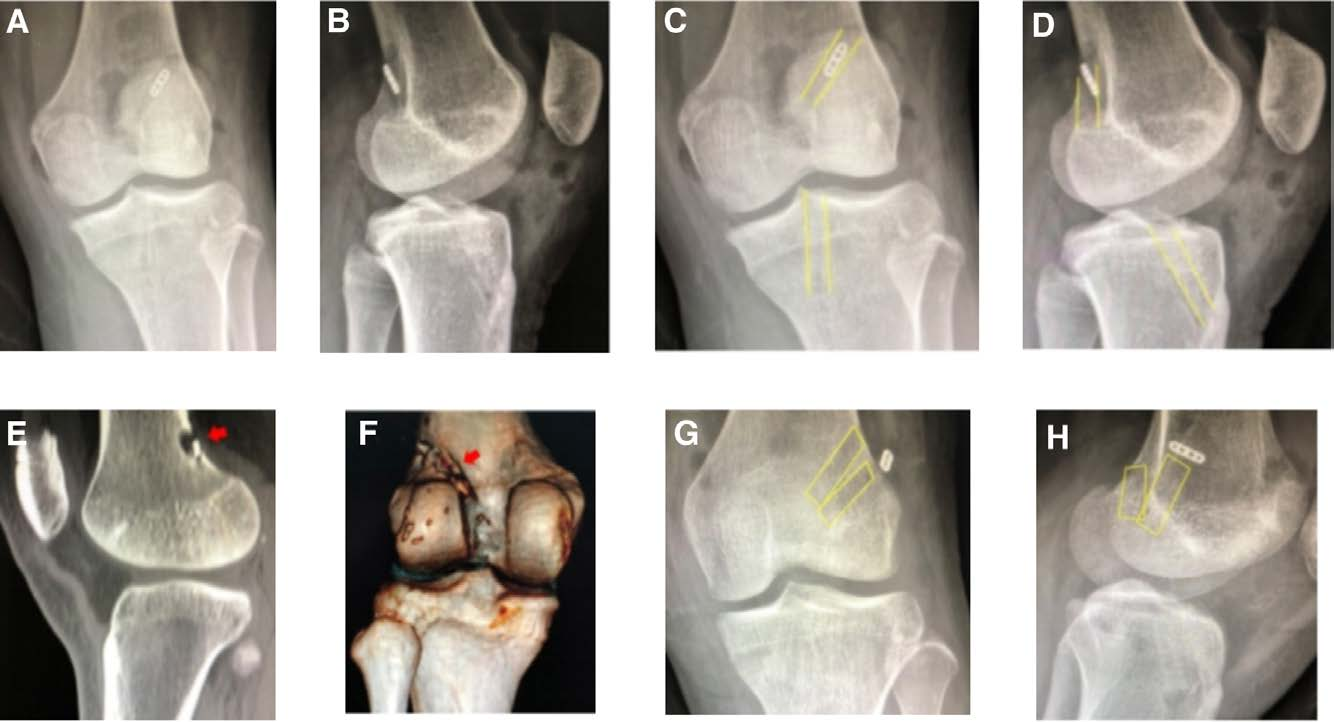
(A and B) DR findings: the femoral suspension EndoButton plate was displaced in the femoral tunnel, and the femoral tunnel was positioned posteriorly. (C and D) The yellow line marks the location of the femoral and tibial tunnel, with the femoral tunnel situated posteriorly. (E and F) CT findings: the femoral suspension EndoButton was displaced in the femoral tunnel, and the posterior wall of the femoral tunnel was broken. (G and H) DR results: the yellow line marks the location of the femoral and tibial tunnel, with the bone canal adjusted anteriorly to maintain the integrity of the posterior wall of the bone canal and increase the length of the bone tunnel.
